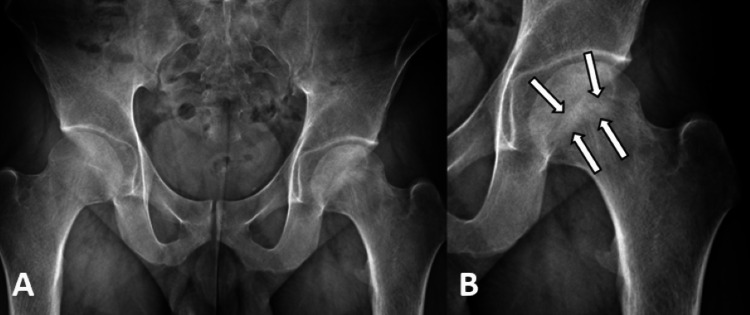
AP plain film of the pelvis (A) and left hip joint (B) Arrows pointing toward a sclerotic band on the left femoral head. AP: Anteroposterior
